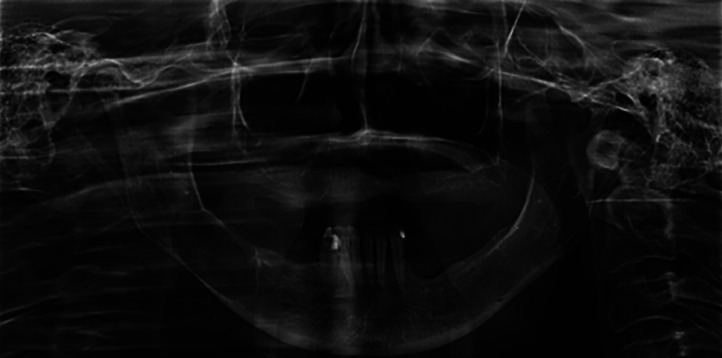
Panoramic radiography showing radiolucent expansile lesion measuring 1.5 cm in diameter located in the right mandibular body.
